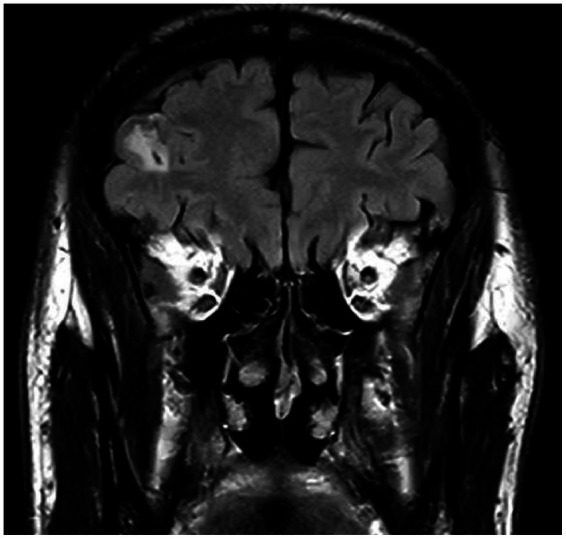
Right frontal lobe lesions demonstrating mild hypointensity on T1-weighted imaging and hyperintensity on T2-weighted/FLAIR sequences, consistent with an infectious process.
